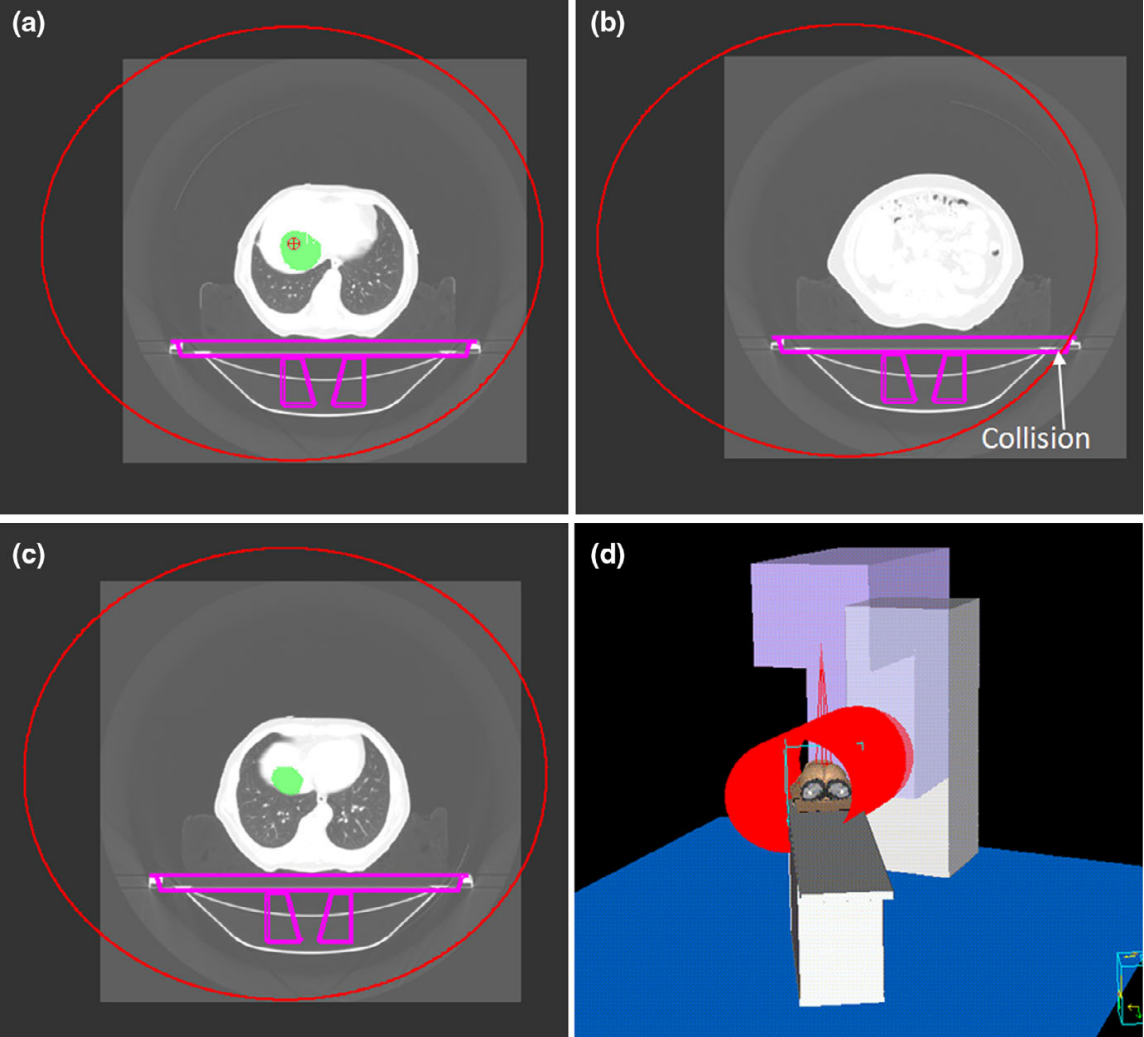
For a liver plan in Pinnacle TPS, ROISS structure is shown as a series of ovals on CT images. (a) is the cross section passing through treatment center, (b) and (c) are the cross sections with off‐center ovals for nonisocenter, (d) shows the three dimensional structure of trajectory ROI in the rooms‐eye view within Pinnacle TPS. The couch angle in this plan is 30°.
